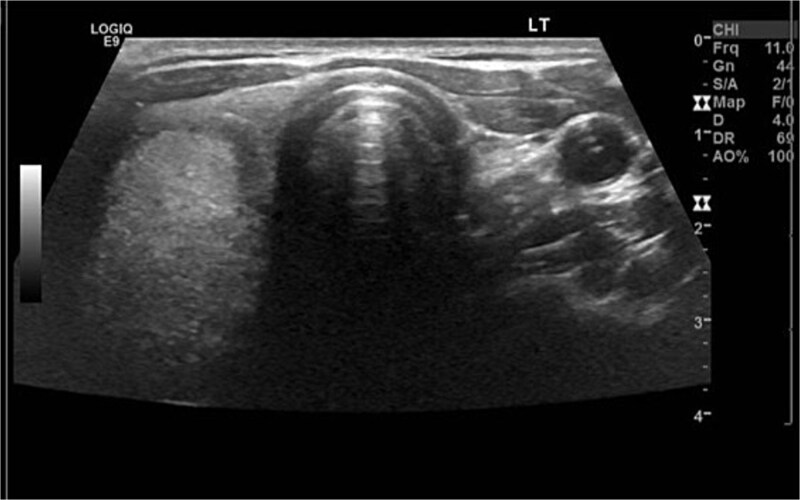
Ultrasound shows the left thyroid lobe is absent.
